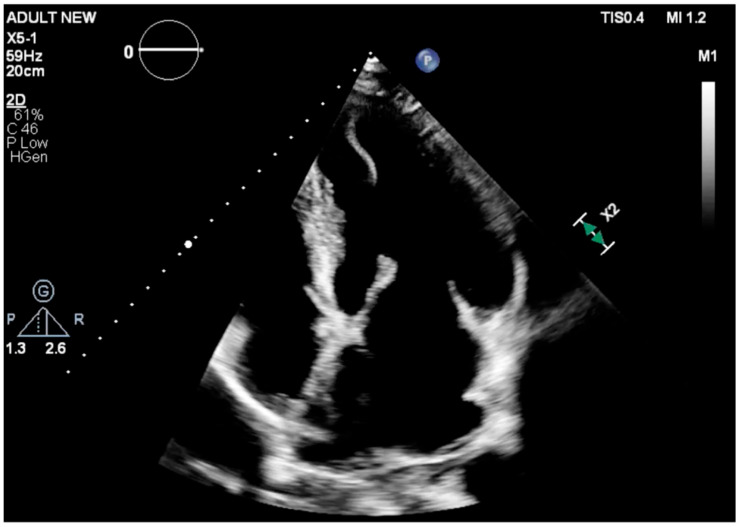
Apical four-chamber transthoracic echocardiographic view revealing a mobile, irregular mass attached to the left ventricular apex.
